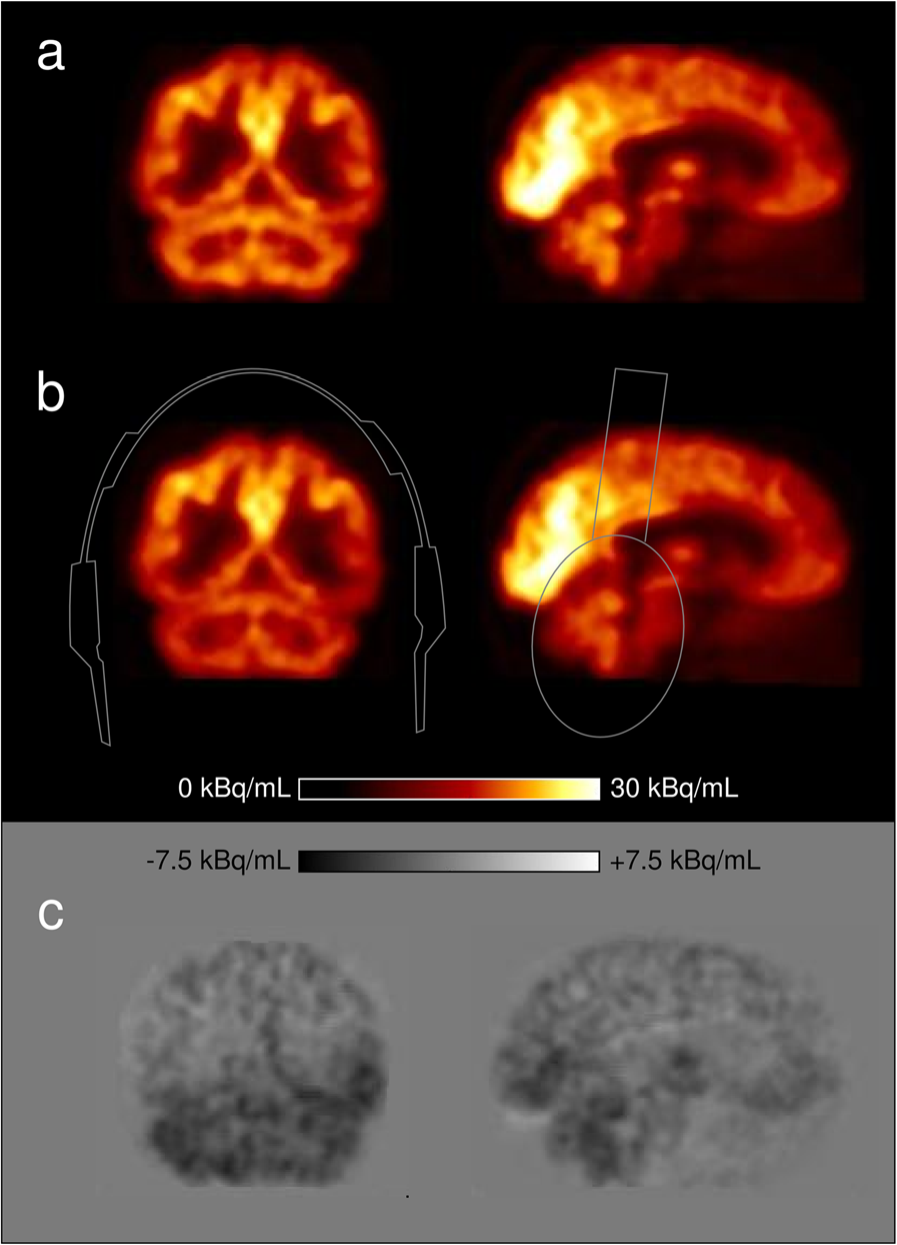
Coronal and sagittal FDG-PET/MR images without (a) and with (b, approximate position indicated by contour) headphones. Difference image (c) reveals a substantial decrease in image values
